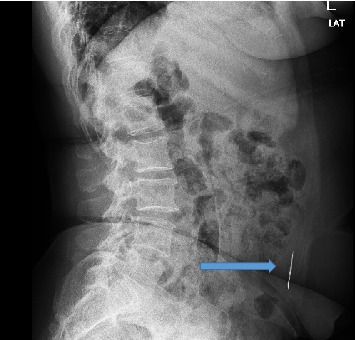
Abdominal X-ray in lateral view showing surgical blade in the abdomen.
